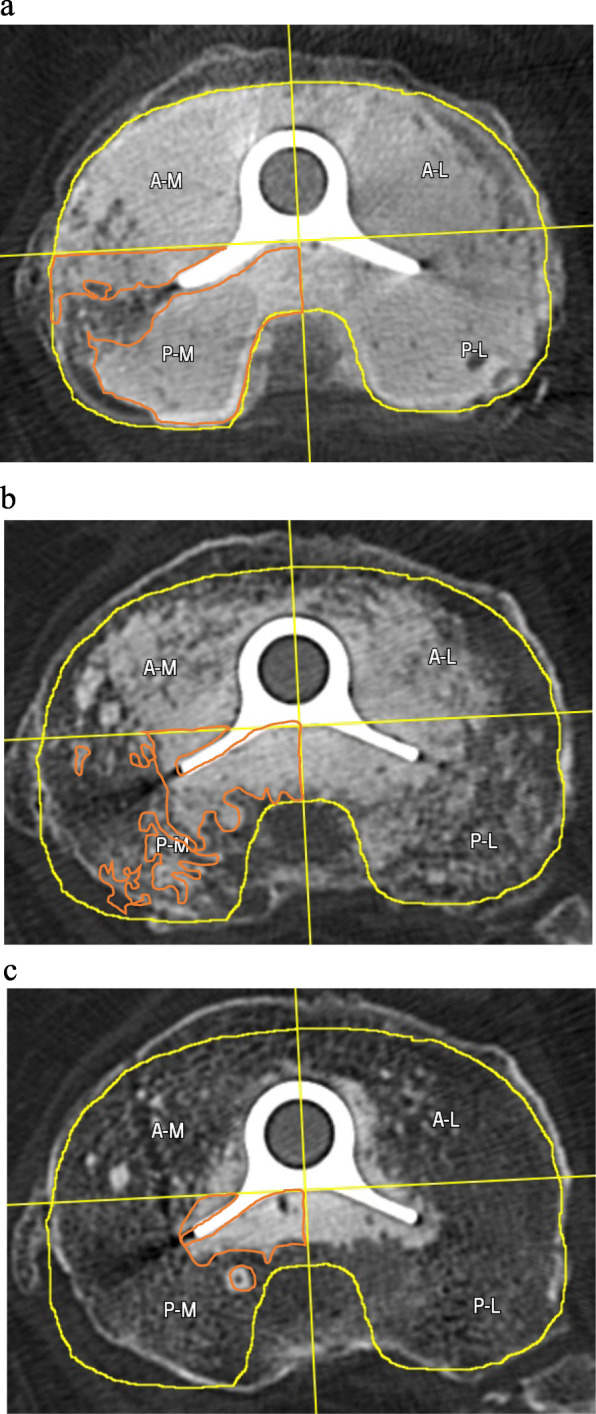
a CT scan 1 mm below the tibial tray. There is an equal distribution of the cement. The yellow line is the projection of the circumference of the tibial tray. The orange line is the circumference of the area with cemented trabecular bone in the Postero-Medial quadrant. A-M = Antero-Medial, A-L = Antero-Lateral, P-M = Postero-Medial, P-L = Postero-Lateral. b CT scan 3 mm below the tibial tray. There is less cement in the periphery of all the three quarters than in the CT slice 1 mm below the tibial tray (Fig. 3a). The yellow line is the projection of the circumference of the tibial tray. The orange line is the circumference of the area with cemented trabecular bone in the Postero-Medial quadrant. A-M = Antero-Medial, A-L = Antero-Lateral, P-M = Postero-Medial, P-L = Postero-Lateral. c CT scan 5 mm below the tibial tray. There is overall less cement in the trabecular bone than in the CT slices 1 mm and 3 mm below the tibial tray. There is less cement in the anterior quadrants than in the posterior quadrants. The yellow line is the projection of the circumference of the tibial tray. The orange line is the circumference of the area with cemented trabecular bone in the Postero-Medial quadrant. A-M = Antero-Medial, A-L = Antero-Lateral, P-M = Postero-Medial, P-L = Postero-Lateral
